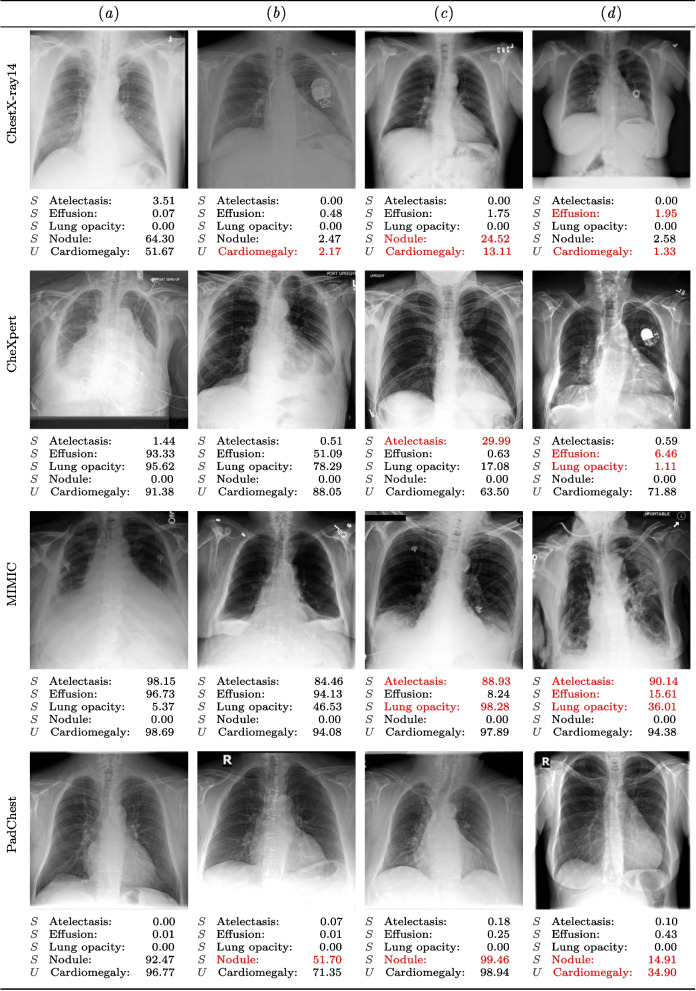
X-ray examples with predicted labels for the 5-way, 1-unseen classification task with 30-shots per class. Each row shows four examples per dataset in MetaChest. Labels below each chest X-ray are annotated with *S* and *U* for seen and unseen classes, respectively. Numbers indicate predicted class probabilities; incorrect predictions are shown in red
